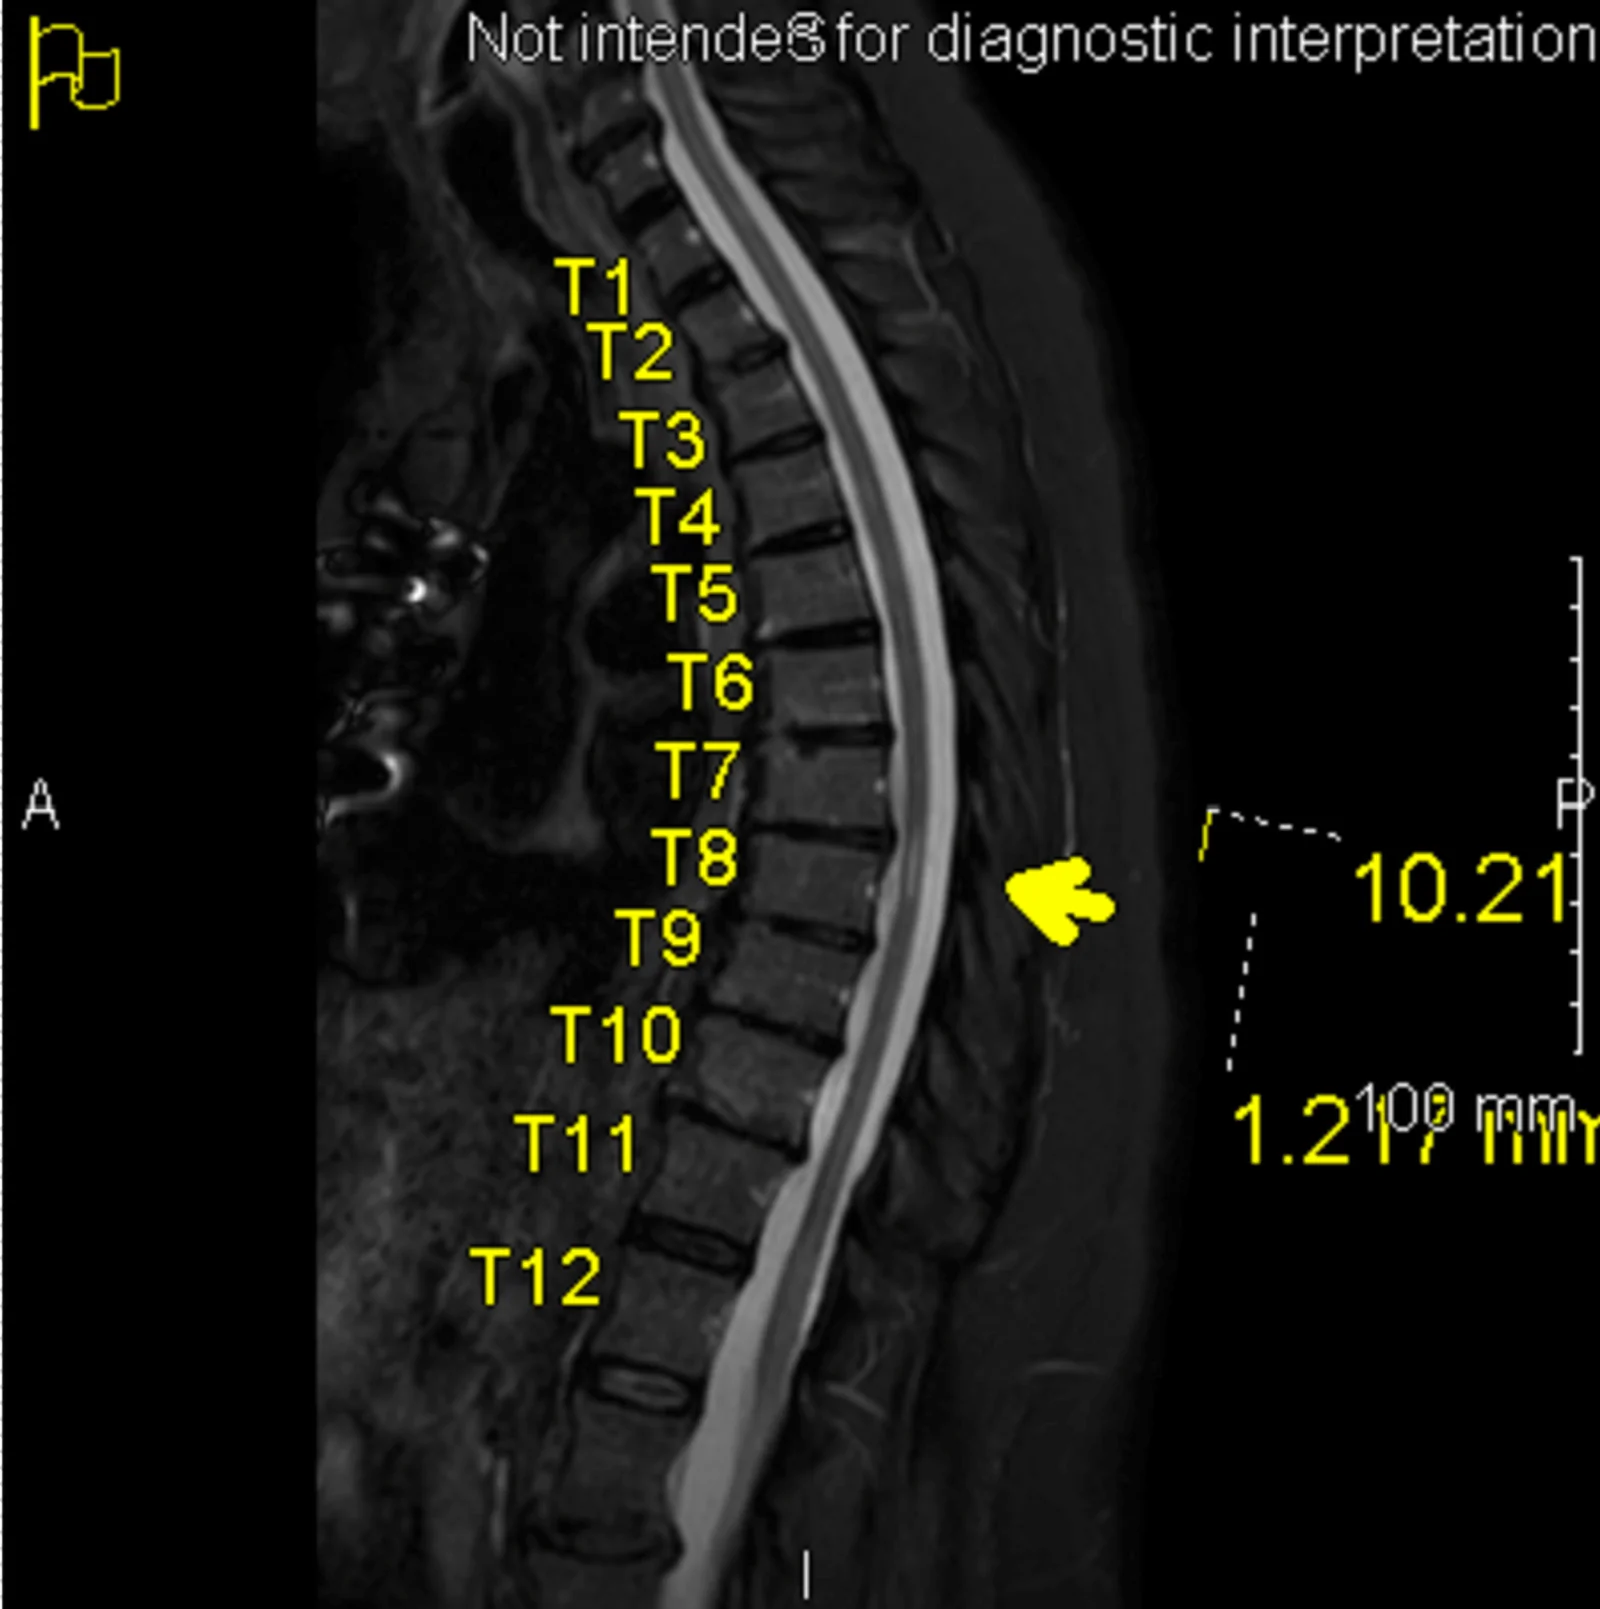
MRI of the thoracic spine (sagittal view). Sagittal view of MRI of the thoracic spine with and without contrast showing linear area of T2 hyperintensity in the thoracic spinal cord at T7-8 through mid T8 level measures up to 10 mm craniocaudal length × 1.2 mm. Slight cord thinning can be noted in this region.
